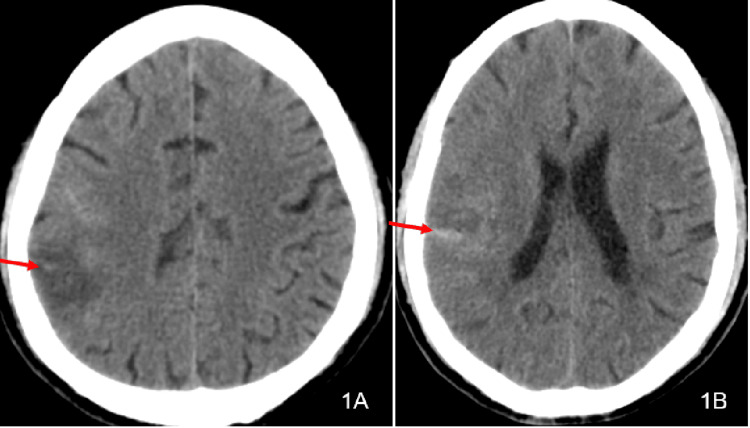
(A-B) Cerebral computed tomography on June 1 indicating right parietal lobe cerebral infarction with minor hemorrhage (arrow indicated)
